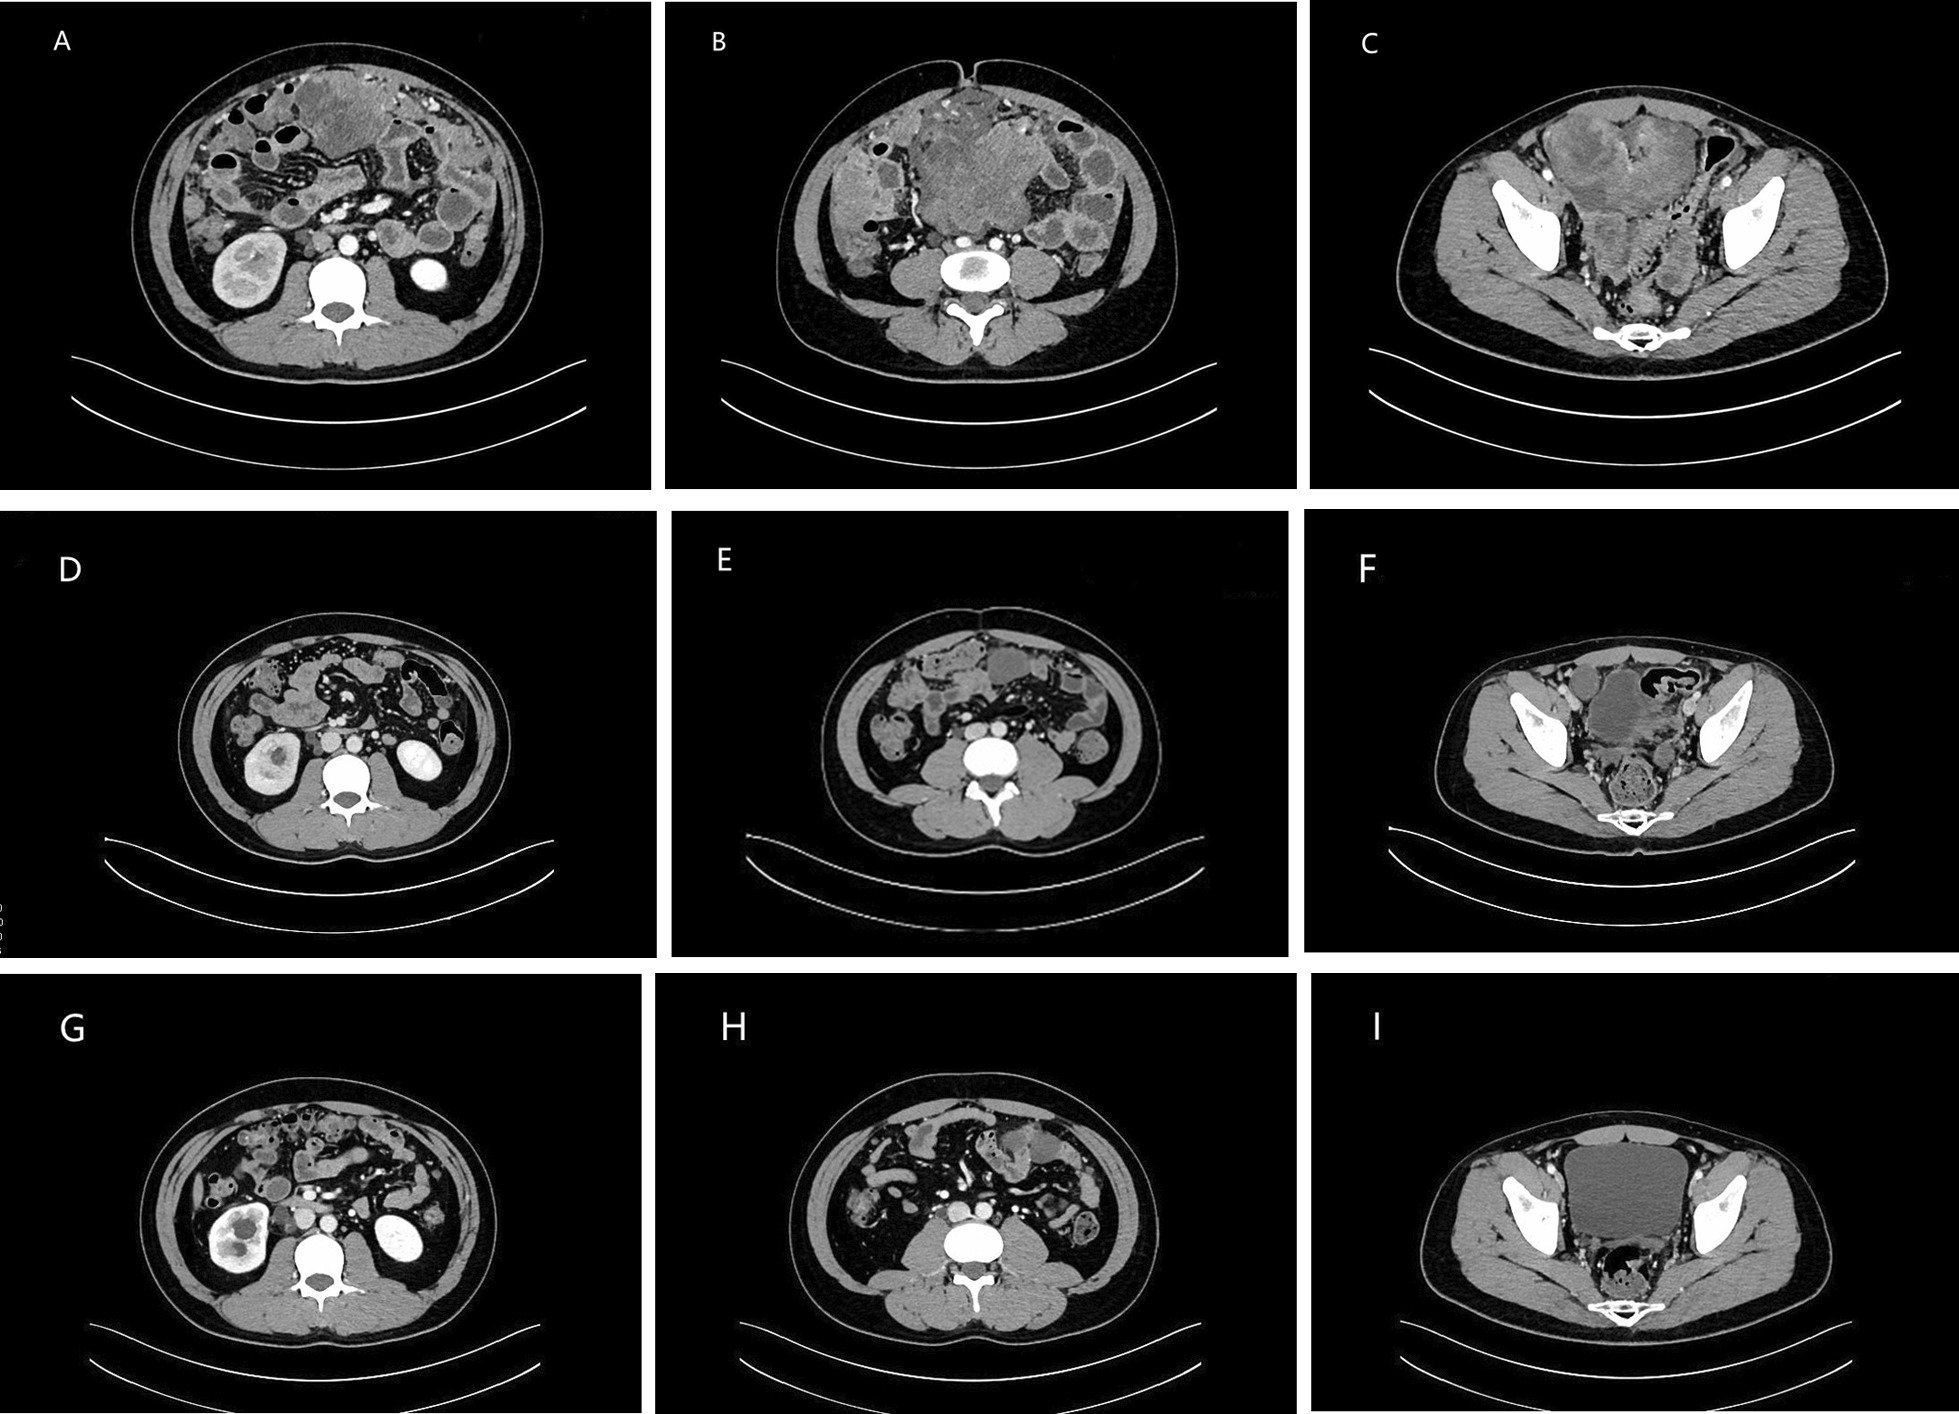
Abdominal CT showing the radiological response to combined and maintenance therapy. A–C Multiple masses in the pelvic and abdominal cavities at the initial diagnosis. D–F Partial response was achieved after two cycles of combined therapy. G–I Continuous partial response during maintenance therapy with anlotinib
